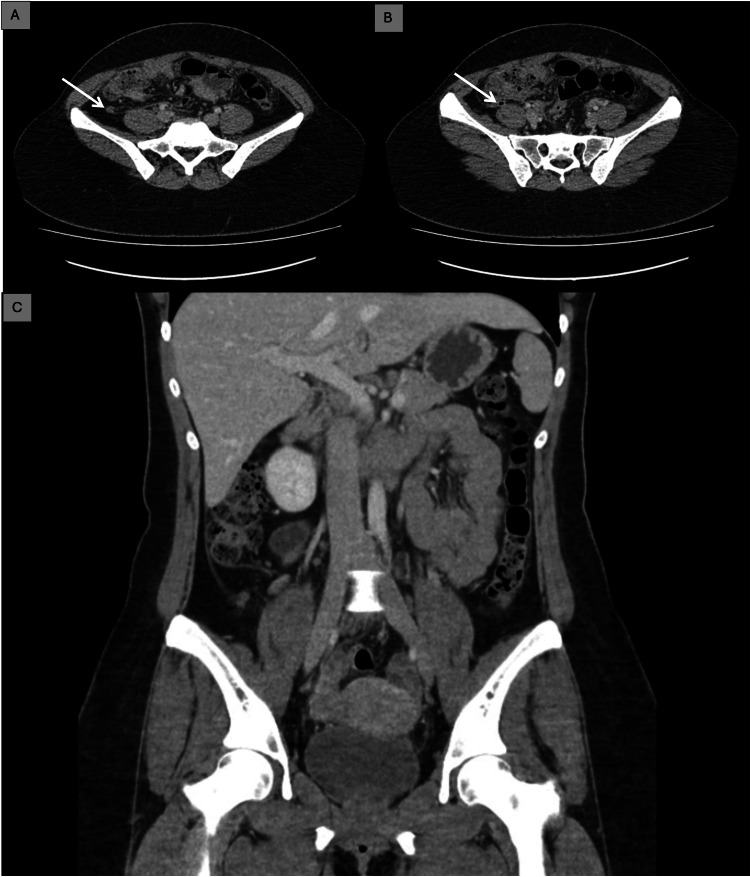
Abdominopelvic CT scan performed in the emergency department. (A, B) Axial views showing findings consistent with appendiceal wall thickening and periappendiceal fat stranding. (C) Coronal view of the pelvic floor without significant findings in the present technique. CT: computed tomography; white arrow: appendix
